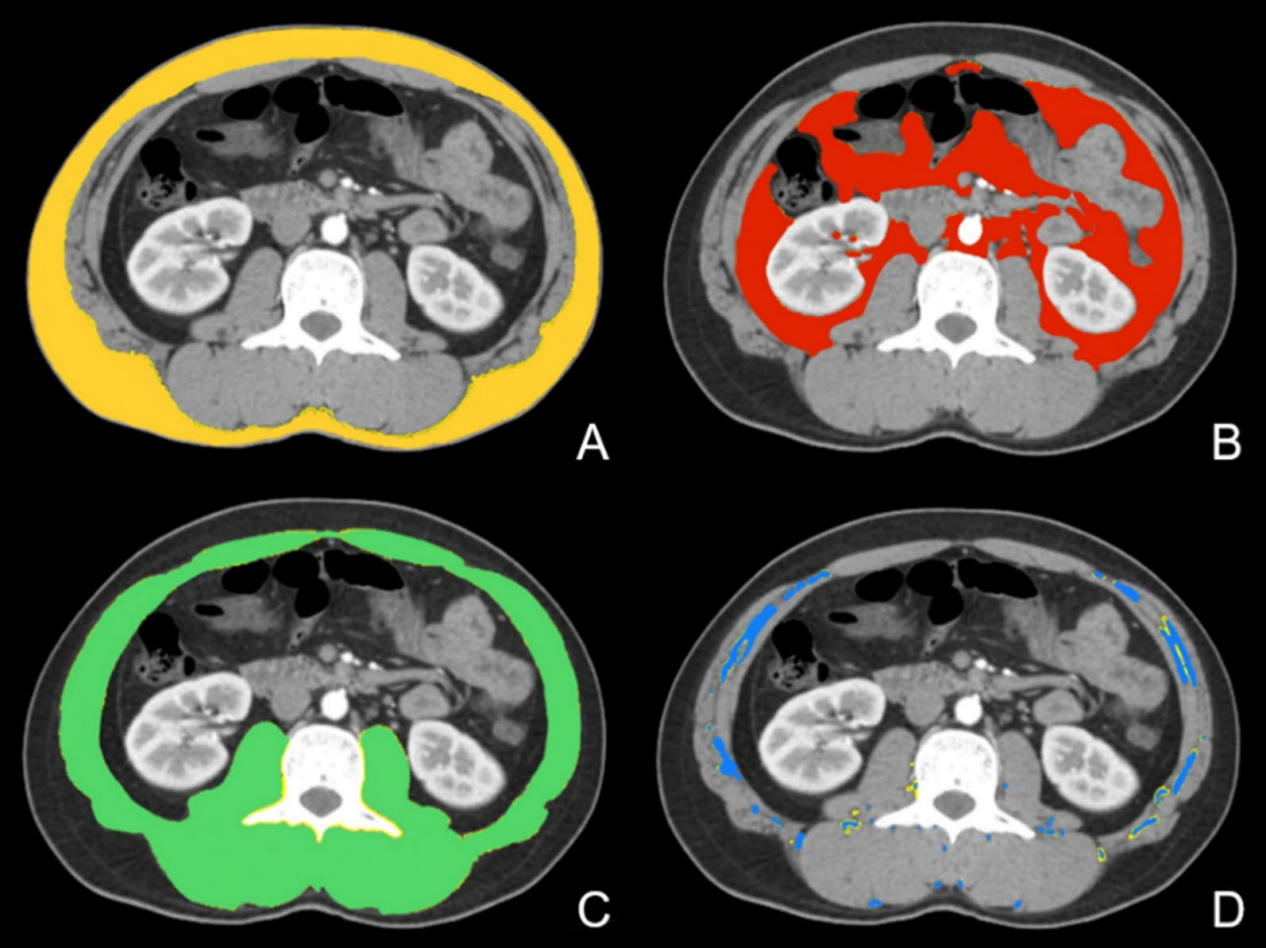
Cross‐sectional computed tomography images at the level of the third lumbar vertebra. A Subcutaneous adipose tissue area (yellow) was quantified using − 190 to − 30 Hounsfield units (HU). B Visceral adipose tissue area (red) was quantified using − 150 to − 50 HU. C Skeletal muscle area (green) was quantified using − 29 to 150 HU. D Intramuscular adipose tissue area (blue) was quantified using − 190 to − 30 HU
